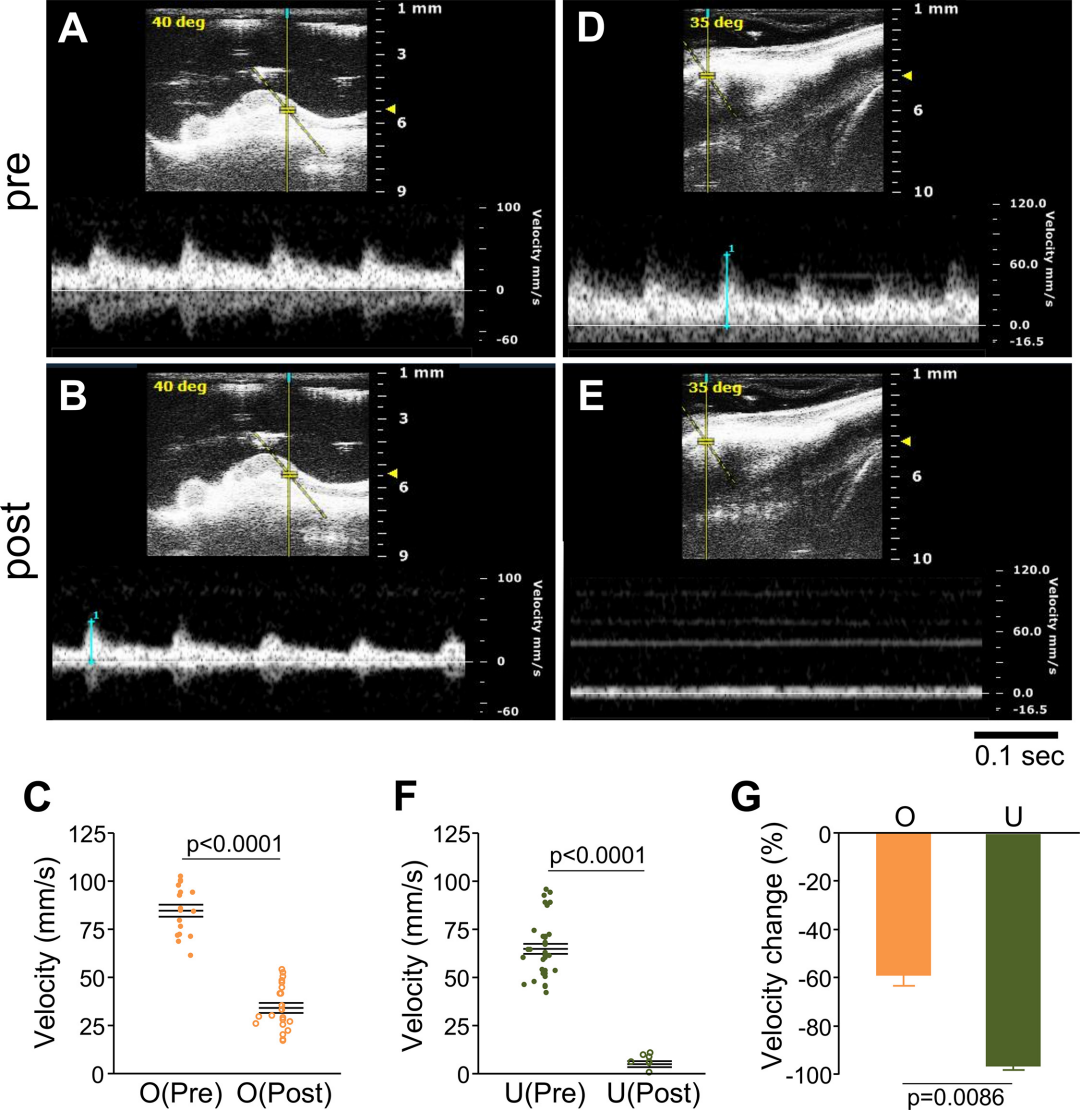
Ligation of uterine vessels reduces arterial blood flow of uterine arcades. Doppler blood velocity waveforms were obtained from an artery of the uterine arcade before (A) or after (B) ligation of ovarian vessels. (C) Summary of arterial blood flow velocity of 4 uterine arcades (4 peaks each) before and after ligation of ovarian vessels. Doppler blood velocity waveforms were obtained from an artery of the uterine arcade before (D) or after (E) ligation of uterine vessels. (F) Summary of arterial blood flow velocity of 5 uterine arcades (4 peaks each) before and after ligation of uterine vessels. (G) Percentage of velocity change relative to velocity before ligation. n = 4 (ovarian vessels) and n = 5 (uterine vessels).
